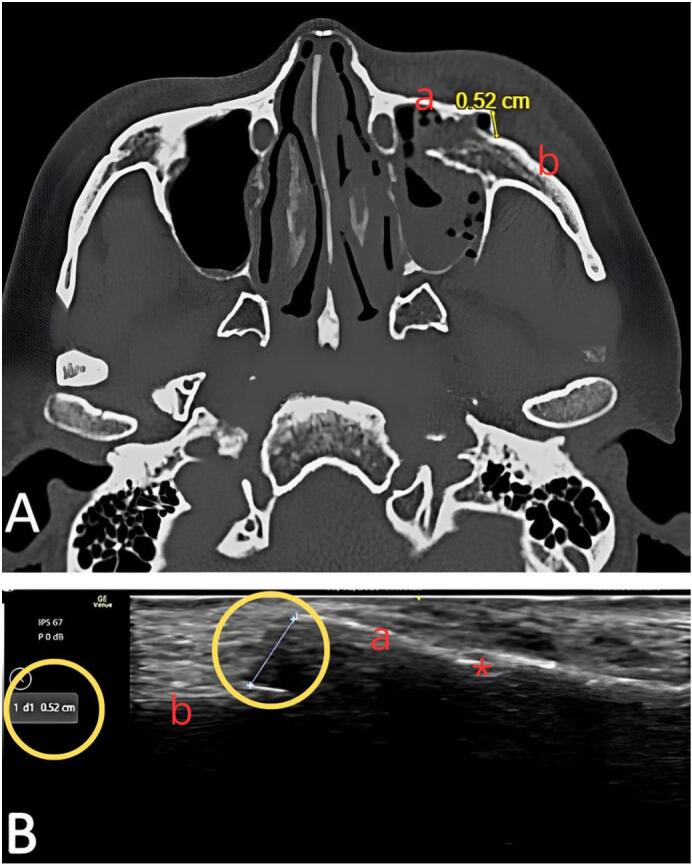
Intraoperative photography. Real time assessment of a zygomatic fracture reduction by ultrasonography. a: J-hook inserted below the body of the zygoma. b: ultrasound probe. c: image visualization.
